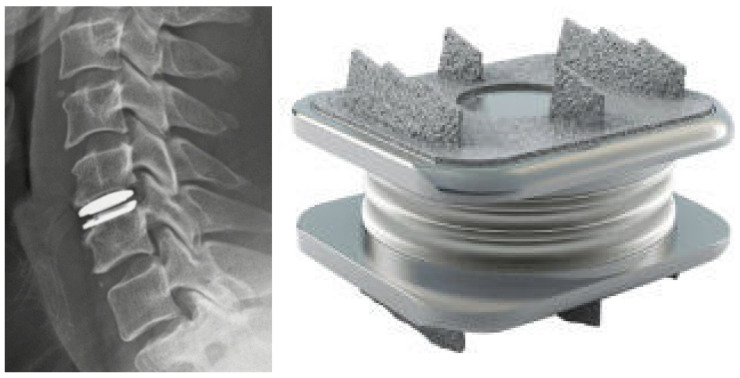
Lateral radiograph of the cervical spine following cervical disc arthroplasty at C5/6 (left). The M6-C^TM^ Artificial Cervical Disc (right).
